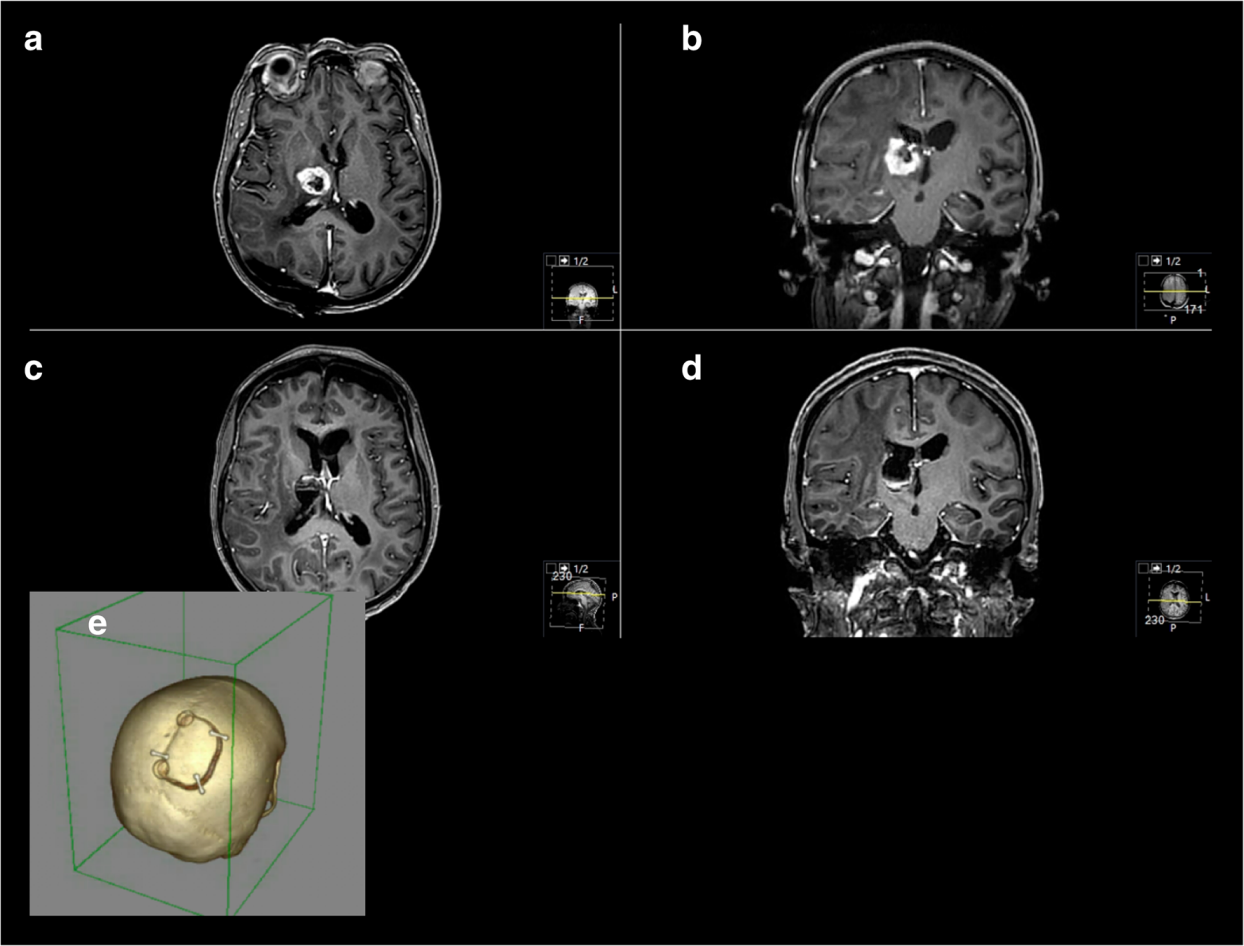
Exemplary case 2: A 52-year-old female patient presented with paresis. Known breast cancer. a, b Preoperative T1-weighted gadolinium-enhanced MR imaging showing a cystic right thalamic tumor. c, d Postoperative MR control showing successful resection. Pathologic findings confirmed the metastasis of breast cancer. e Postoperative 3D-CT reconstruction showing the bone flap for a PIA
